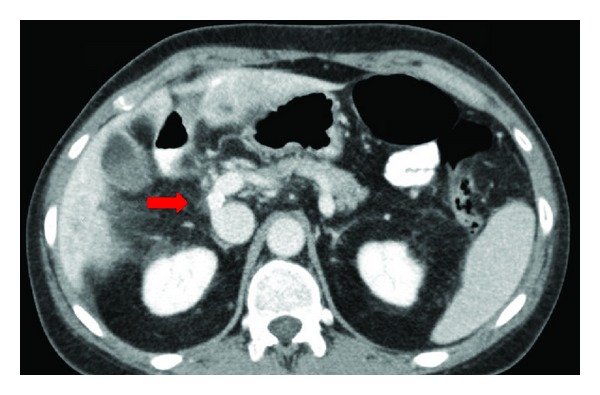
CT abdomen with IV contrast two weeks following surgical portacaval shunt placement. An 8 mm polytetrafluorethylene (PTFE) graft connects the main portal vein with infrahepatic inferior vena cava (red arrow).
